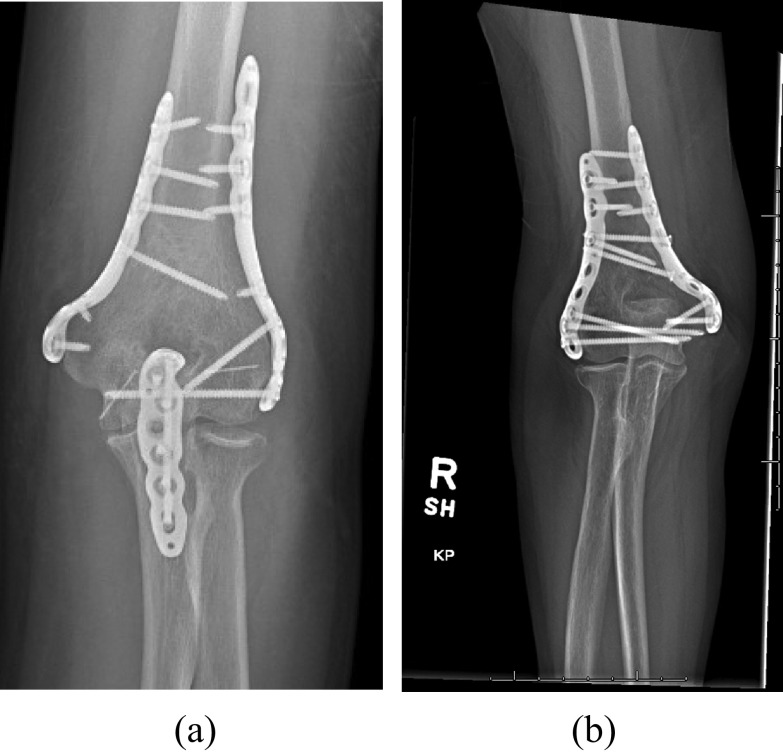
(a) AP radiograph 2 years post-op showing placement of 2 screws in distal fragment. Parallel plating of the distal humerus shaft is also shown in the image. There is osseous bridging across a transfixed olecranon osteotomy. (b) AP radiograph 2 years post-op showing articular reduction and complete bony healing of distal humerus fracture. The repaired construct included parallel plating with 5 articular fragment locking screws with interdigitation between the medial and lateral plate screws.
